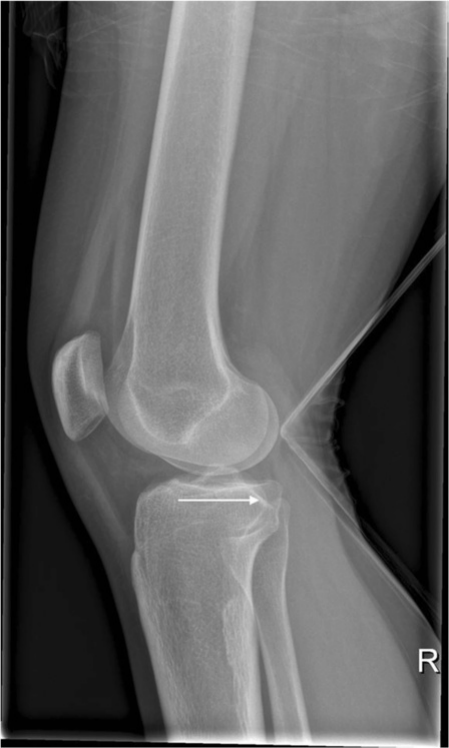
Lateral X-ray view of a tibial plateau fracture (Schatzker II) shown in Fig. 1
